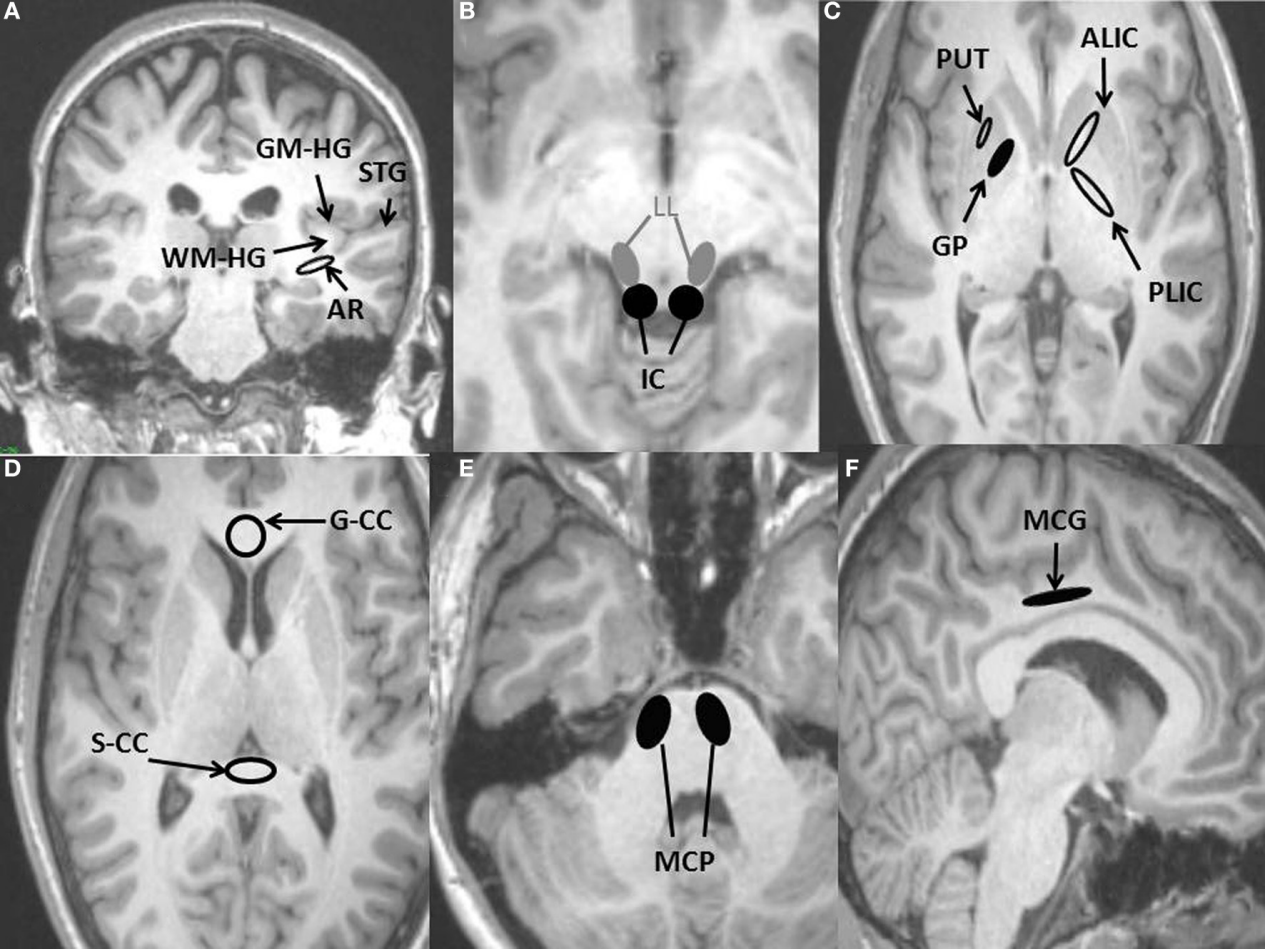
Regions of interest (ROIs) used for DTI measurements placed on anatomical T1-weighted MRI scan. (A) Four auditory ROIs, Gray matter of Heschl's gyrus (GM-HG), White matter of Heschl's gyrus (WM-HG), Superior temporal gyrus (STG), and Auditory radiation (AR). (B) Two auditory ROIs, Inferior colliculus (IC), Lateral lemniscus (LL). (C) Three non-auditory ROIs, Putamen (PUT), Globus pallidus (GP), Posterior limb of the internal capsule (PLIC), Anterior limb of internal capsule (ALIC). (D) Two non-auditory ROIs, Genu of the corpus callosum (G-CC), Splenium of the corpus callosum (S-CC). (E) Non-auditory ROI, Middle cerebellar peduncle (MCP). (F) Non-auditory ROI, Middle cingulate gyrus (MCG).
